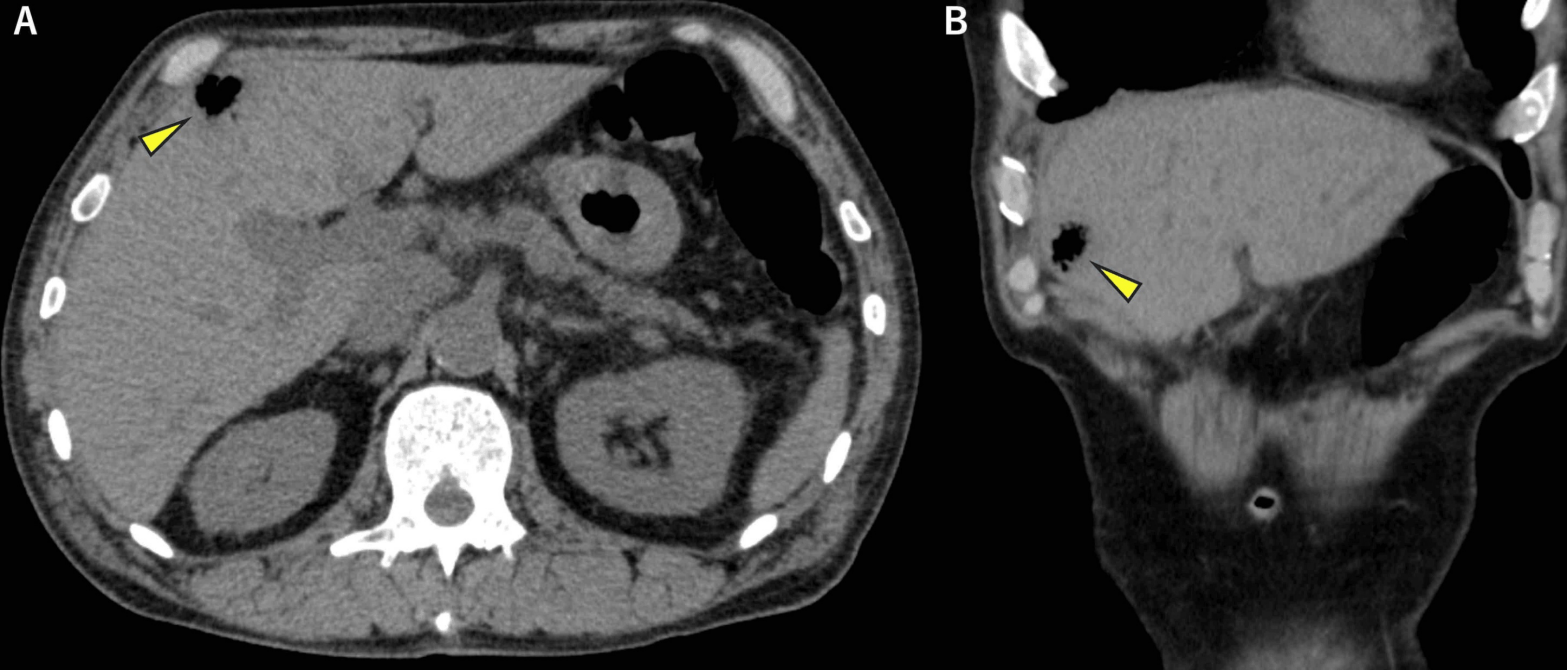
Abdominal non-contrast CT (A, B) Abdominal non-contrast CT revealed gas formation in liver segment Ⅳ, measuring up to 17 mm in diameter (arrowheads).
